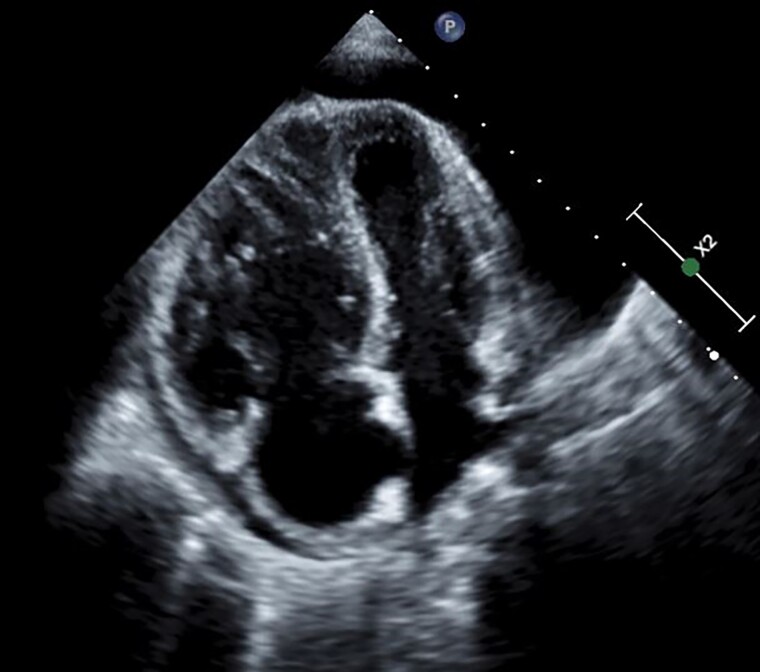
Transthoracic echocardiography (apical four-chamber view) showing large pericardial effusion and dilated hypertrophied right ventricle.
